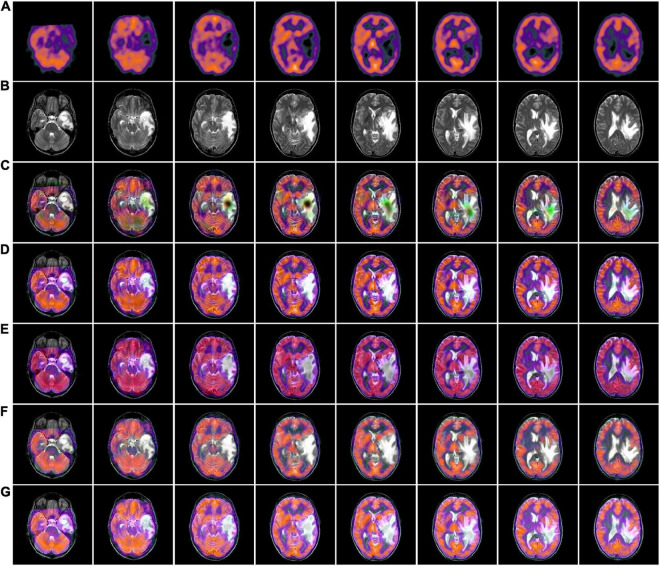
MRI-SPECT fusion images of the five methods in Paras2. (A) Single photon emission computed tomography (SPECT) source image; (B) magnetic resonance imaging (MRI) source image; (C) nonsubsampled contourlet (NSCT) fusion result; (D) non-subsampled shear wave transform (NSST) fusion result; (E) guided filter ng fusion (GFF) fusion result; (F) Laplacian redecomposition (ReLP) fusion result; (G) fusion result of the proposed method.
